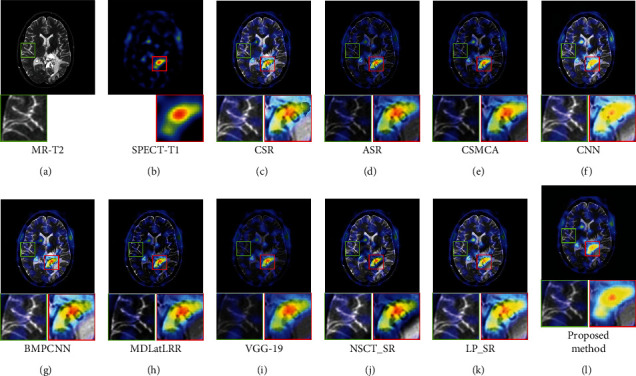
Comparison of the fusion results in the second set images. (a) MR-T2. (b) SPECT-T1. (c) CSR. (d) ASR. (e) CSMCA. (f) CNN. (g) BMPCNN. (h) MDLatLRR. (i) VGG-19. (j) NSCT_SR. (k) LP_SR. (l) Proposed method.
